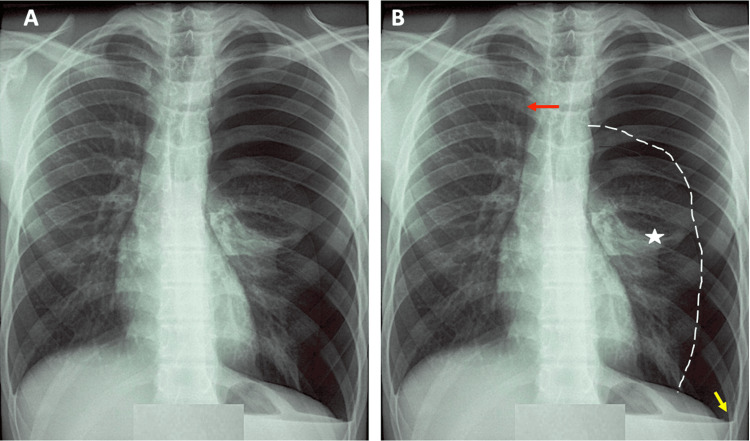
Frontal chest radiograph (A) A large avascular hyperlucent area occupies nearly the entire left hemithorax, consistent with a complete left-sided pneumothorax. (B) The left lung is completely collapsed and retracted toward the mediastinum (white star), delineated by a fine white pleural demarcation line. Blunting of the left costophrenic angle is observed (yellow arrow), suggesting associated pleural thickening or effusion. The trachea is deviated to the right (red arrow), indicating a mass effect consistent with tension physiology. The right lung appears radiographically normal. These findings are diagnostic of a compressive total left pneumothorax.
